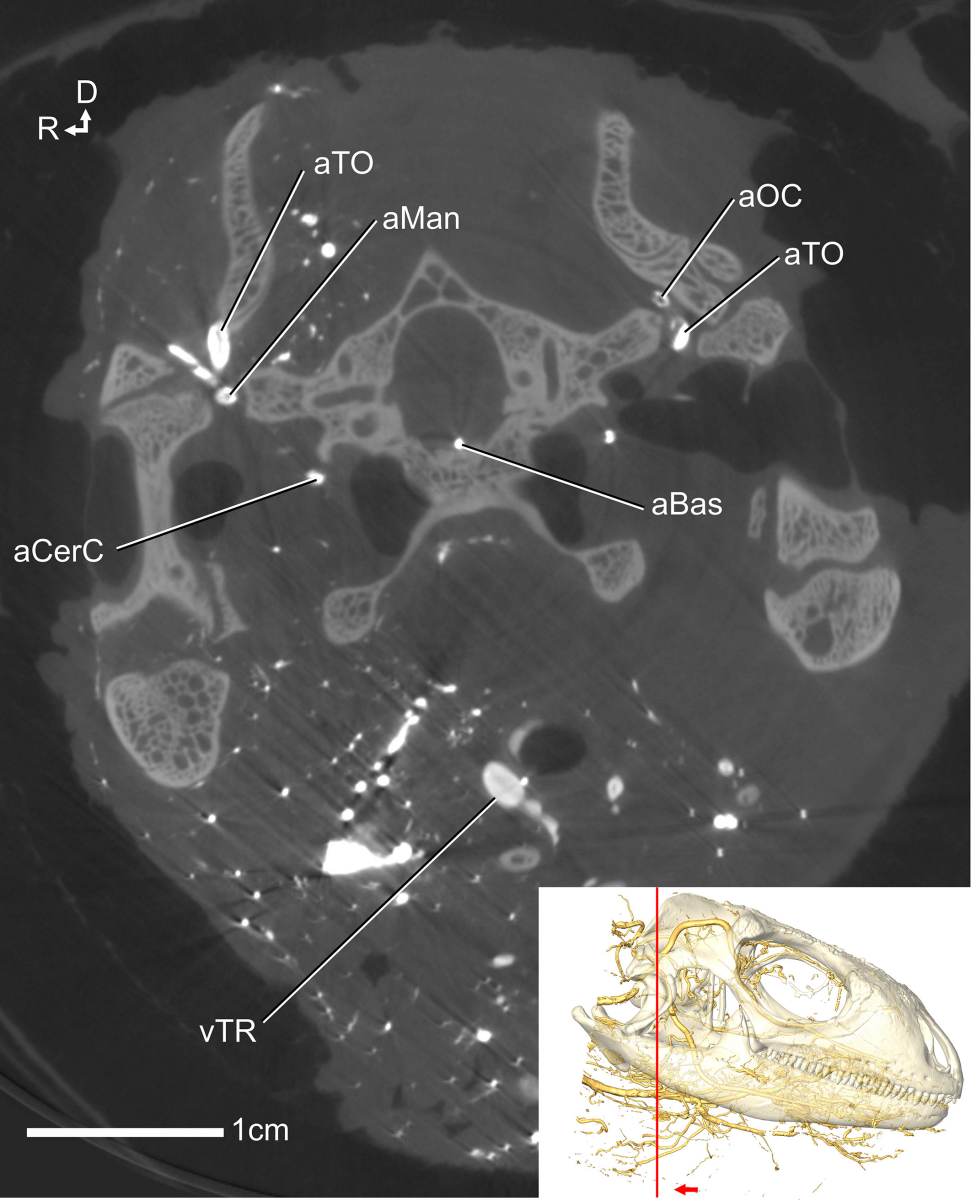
CT slice of *Iguana iguana* OUVC 10611 showing arteries and veins using the differential contrast dual vascular injection technique. Veins were injected with the solution containing less barium, resulting in a less bright region in the CT scan slice, see vTR. The arteries were injected with a greater concentration of barium, resulting in a brighter region in the CT scan slice, see aTO. D indicates dorsal and R indicates right side. Inset showing transparent skull and vascular isosurface rendering indicates slice location and orientation.
